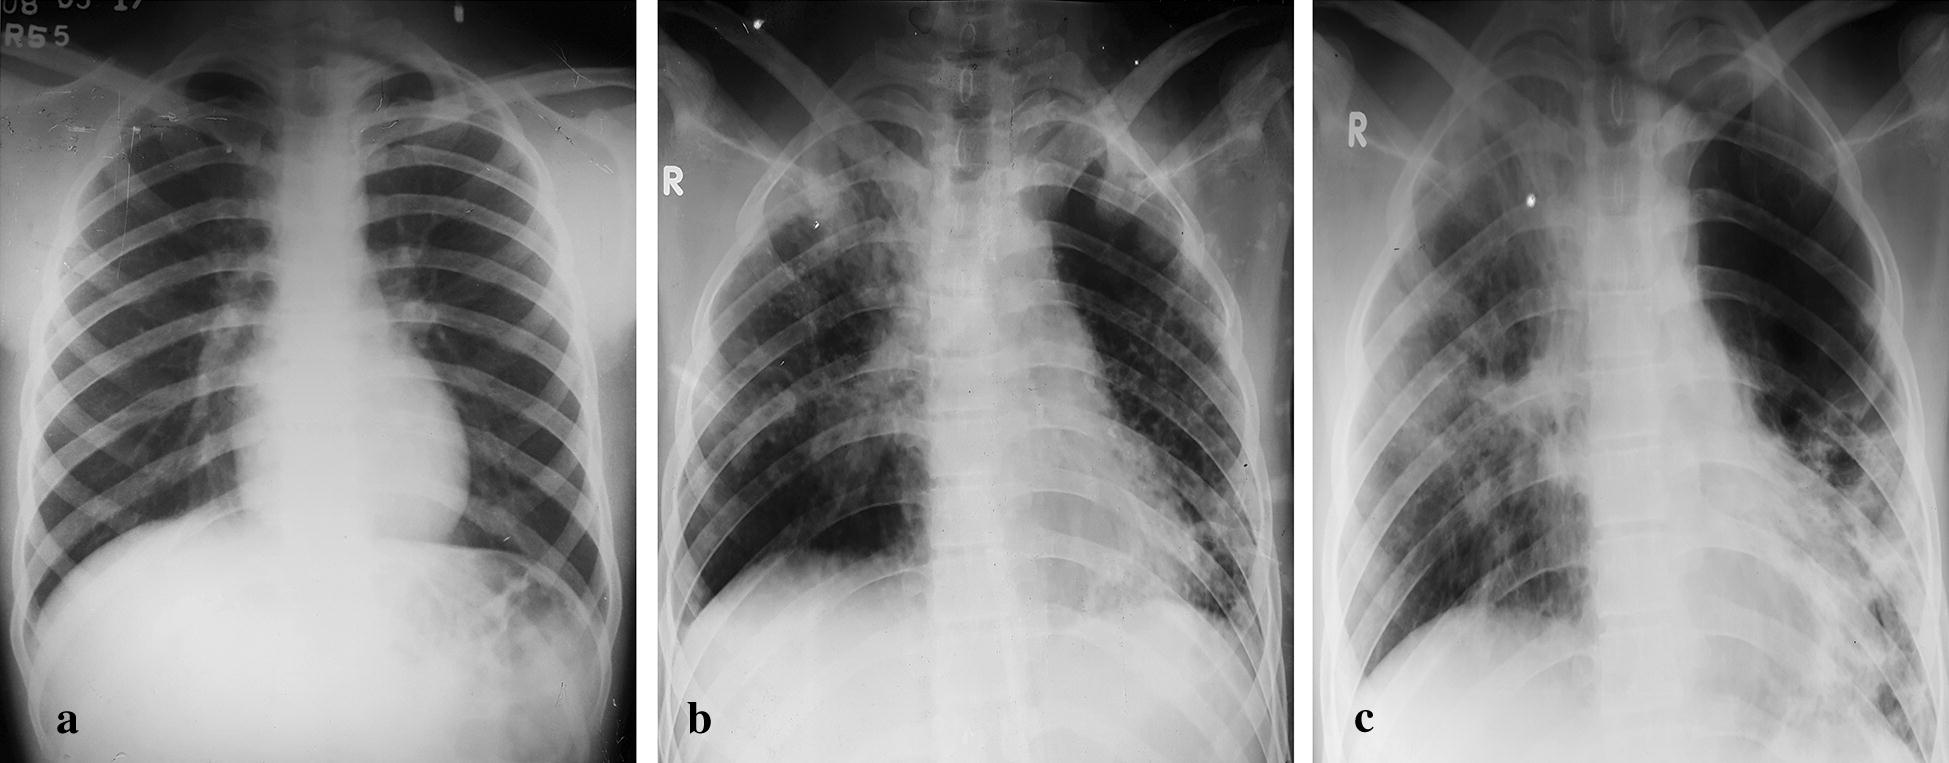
Serial chest radiographs after Paraquat poisoning. a Day 1—Normal. b Day 15—Diffuse alveolar shadowing predominantly involving left mid and lower zone. c Day 30—Diffuse alveolar shadowing extending to right apical and mid zone
